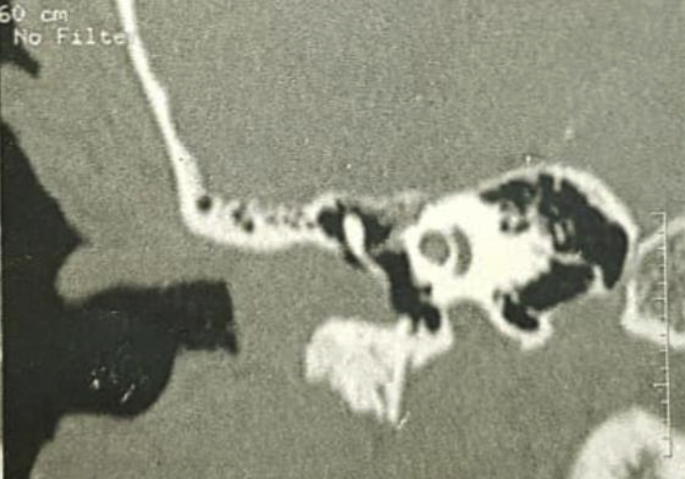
CT image showing a tissular tumor of the EAC. The middle ear and the temporal bone are intact.
